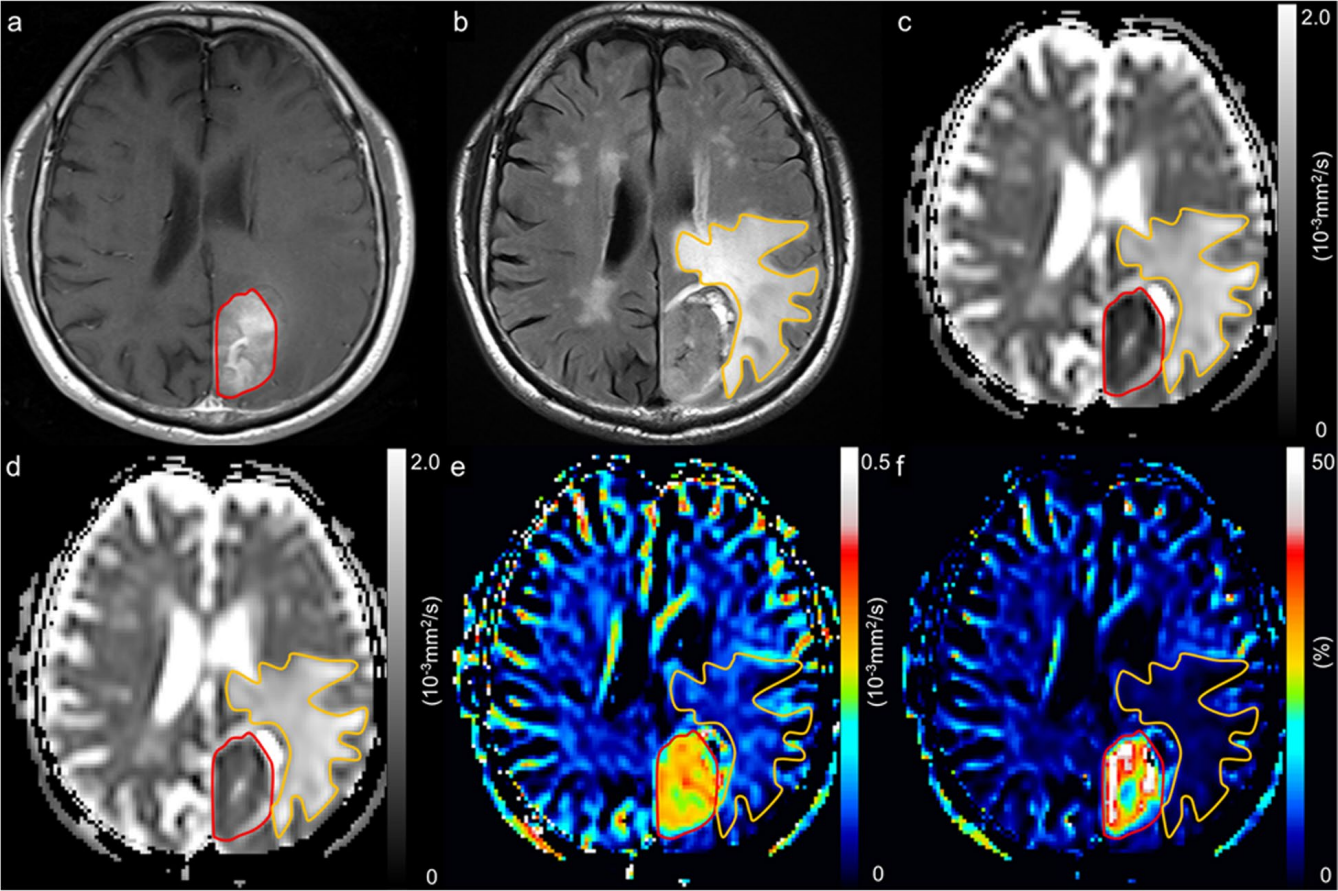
A 69-year-old man with a brain metastasis from colon cancer. A contrast-enhanced T1-weighted image with a region of interest of the enhancing region (red line) (a), a FLAIR image with a region of interest of peritumoral region (orange line) (b), an apparent diffusion coefficient (ADC) map derived from pulsed gradient spin-echo (PGSE) DWI at an effective diffusion time (Δ_eff_) of 44.5 ms (c), an ADC map derived from oscillating gradient spin-echo (OGSE) DWI at an Δ_eff_ of 7.1 ms (d), and maps of ADC change between PGSE DWI and OGSE DWI (cADC) (e) and relative ADC change between PGSE DWI and OGSE DWI (rcADC) (f). The ADC values in the tumor appear higher at short Δ_eff_ values than at long Δ_eff_ setting. Large changes in cADC and rcADC are noted between the OGSE and PGSE sequences in the tumor
